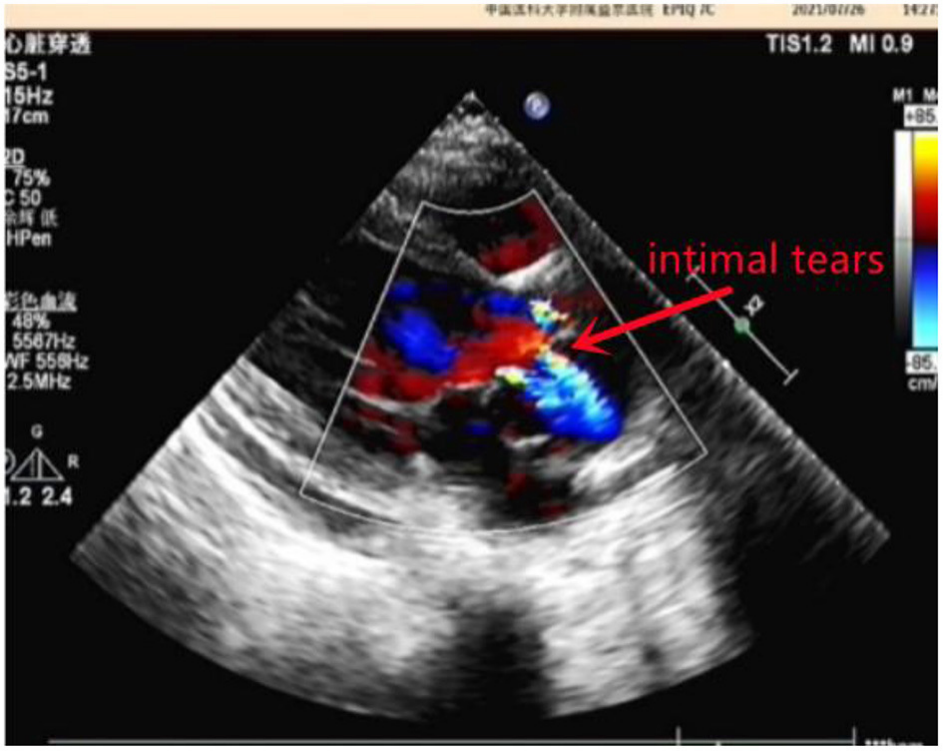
Color Doppler echocardiography.
